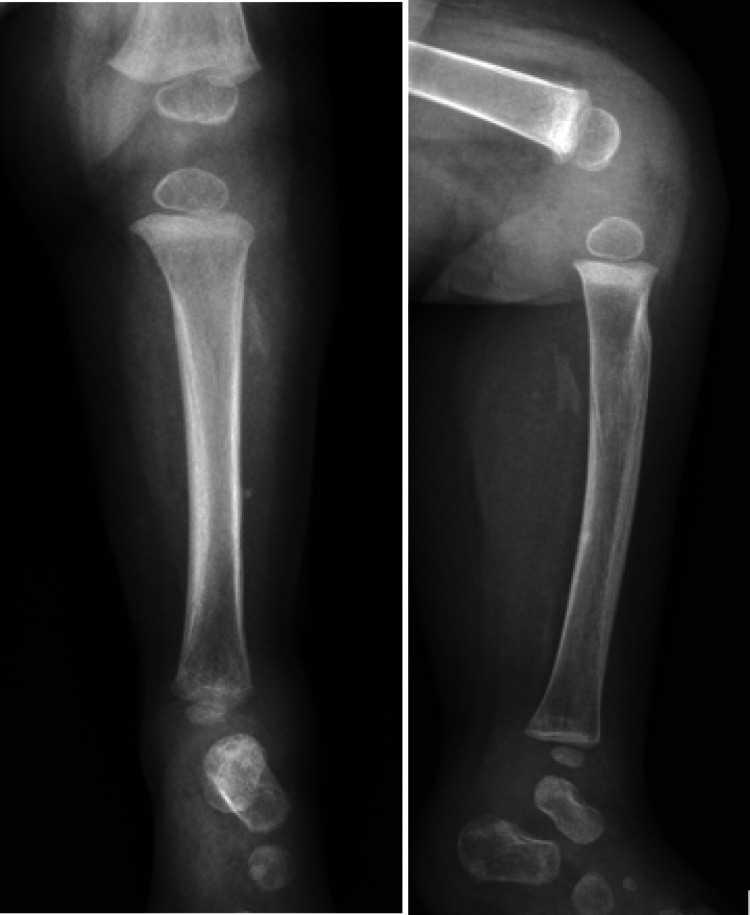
Radiography of the left leg after resection Anteroposterior (Panel A) and lateral (Panel B) of the left lower extremity after fibular resection.
